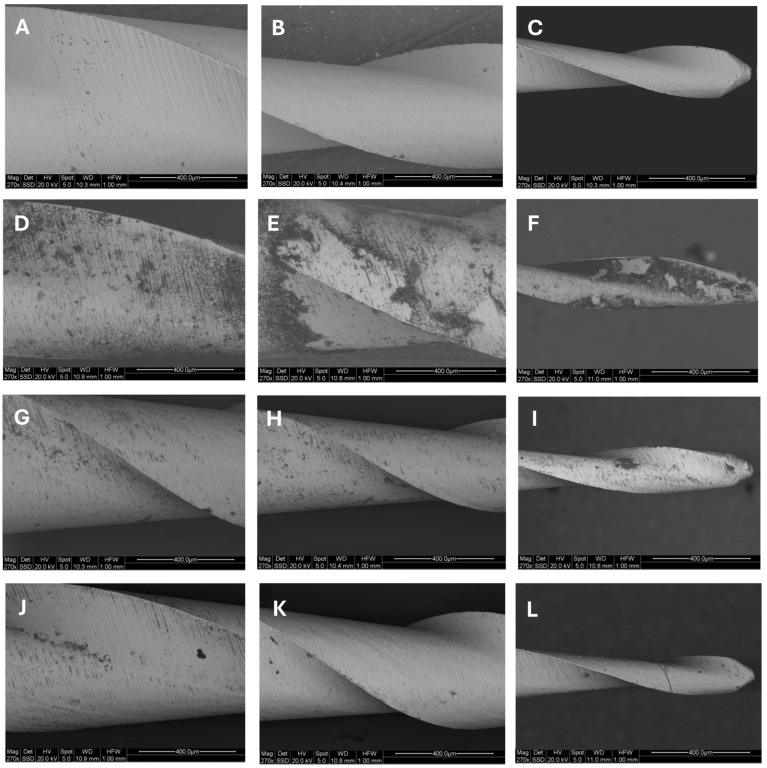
Representative images of SEM back-scattered acquisitions at 270× magnification of instruments’ sections from Group I (new instruments; (A) coronal, (B) middle, and (C) apical), Group II (uncleaned, autoclaved instruments; (D) coronal, (E) middle, and (F) apical), Group III (ultrasonic cleaning and steam sterilization; (G) coronal, (H) middle, and (I) apical), and Group IV (scouring sponge, ultrasonic cleaning, and steam sterilization; (J) coronal, (K) middle, and (L) apical).
